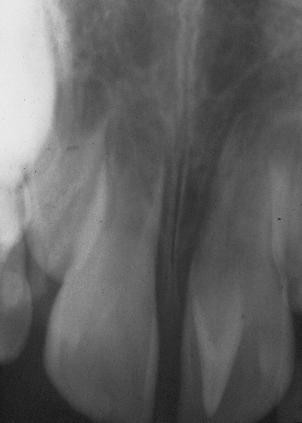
Talon cusp radiograph. Periapical radiograph of upper central incisor showing a V-shape structure superimposed over the normal image of the crown
